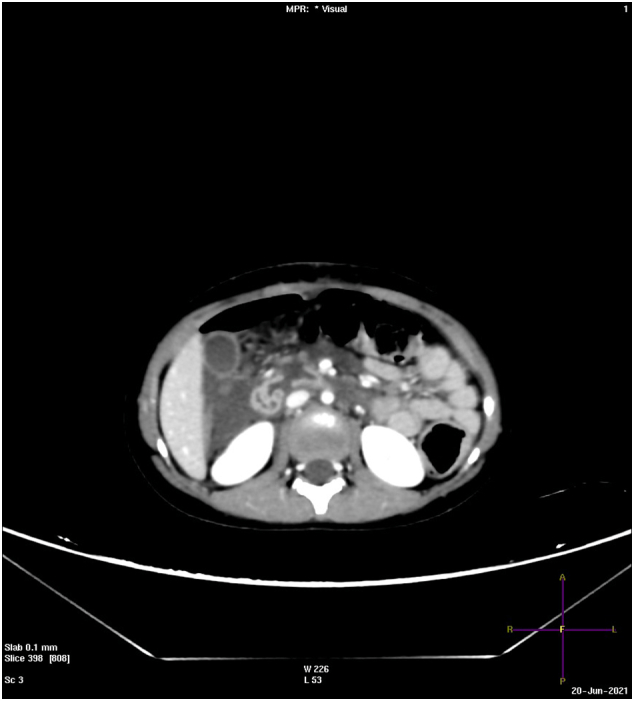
Axial CT with IV contrast; perforation seen in 3rd part of duodenum with free fluid.
